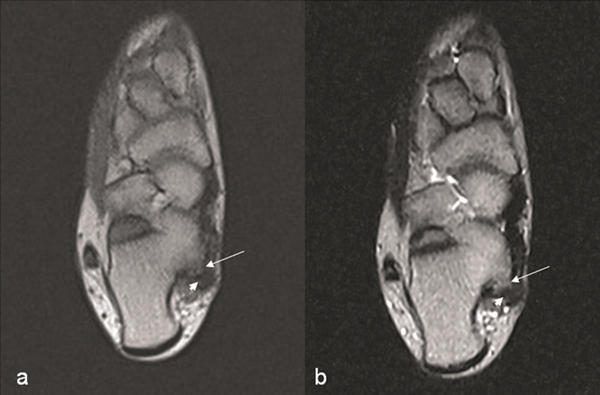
Axial T1-weighted (A) and T2-weighted (B) MR images taken at the 6-month the first-operative of the foot shows an intermediate signal intensity lesion (arrow) in contact with the calcaneus. The cartilage cap has shown the low signal intensity in image both T1-weighted and T2-weighted MR (arrowhead: cartilage cap).
